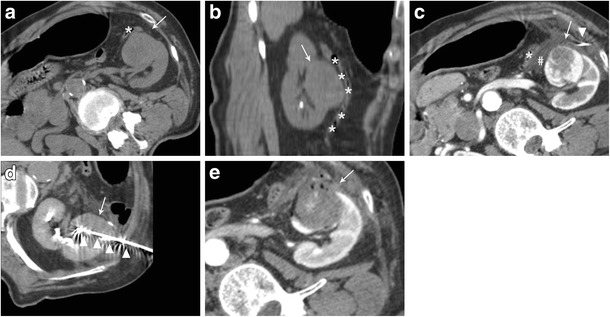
Treatment of an anterior left renal tumour in close proximity with a bowel loop. a CT performed the day of treatment showing the close proximity of the tumour to be treated (white arrow) with a bowel loop (asterisks). Note the external compression to obtain the desired position of the patient for an easy access to the target tumour. b multiplanar reconstruction of the CT images showing the close proximity of the tumour (white arrow) with a bowel loop (asterisks). c A CT scan performed after fluid (*hash*) injection confirmed the correct displacement of the colon (asterisks) from the target tumour (*white arrow*) (*arrowhead = spinal needle*). d CT scan demonstrating the insertion of the microwave antenna (*arrowhead*) into the renal tumour (*white arrow*). e Contrast-enhanced CT performed immediately after treatment demonstrating the complete ablation of the renal tumour (*white arrow*) without complications
